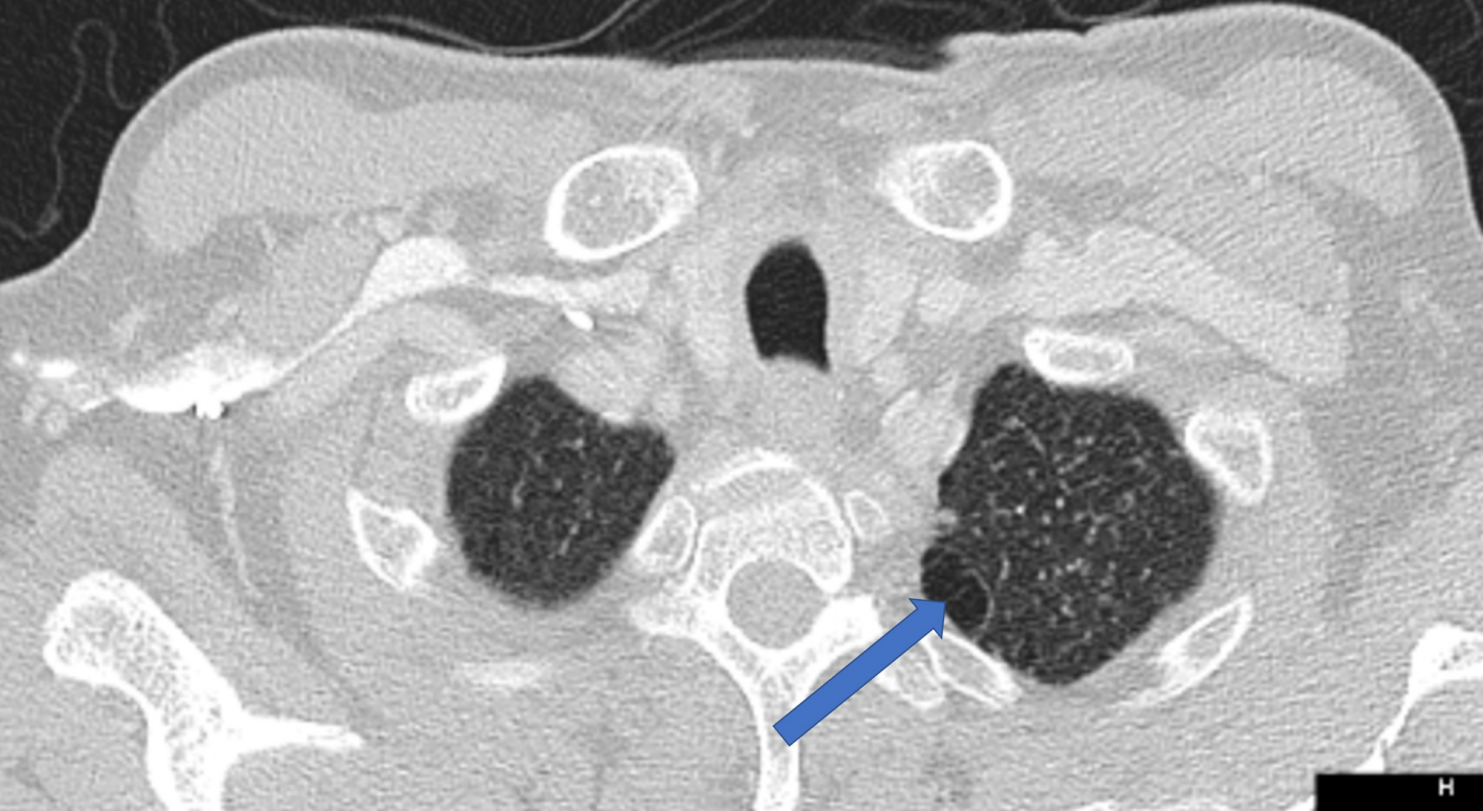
CT scan of chest on initial admission Pulmonary nodule seen in the left lobe of the lung (arrow)
